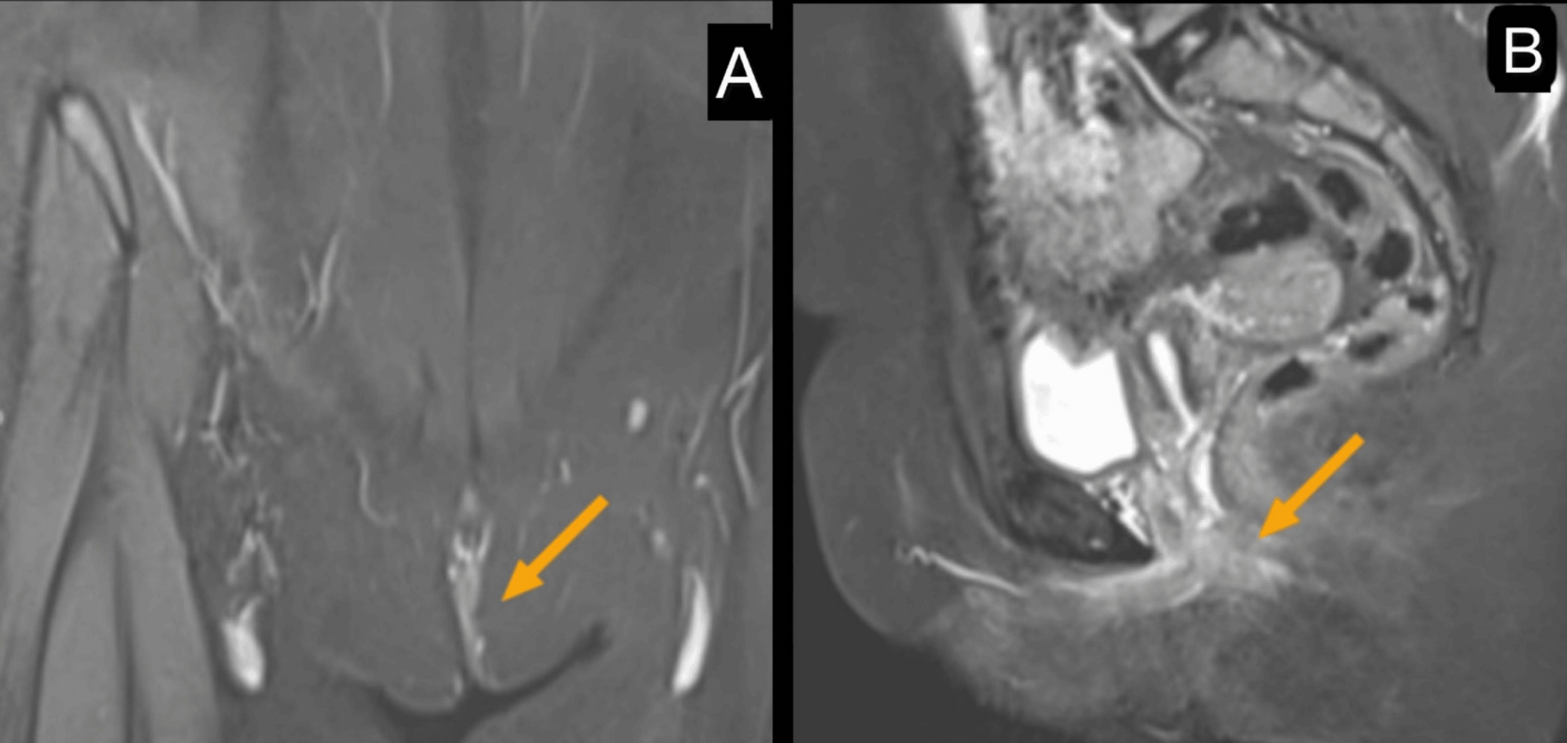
MRI fistulogram shows a curvilinear sinus tract with surrounding fibrotic changes in the subcutaneous plane of the right perineum (yellow arrow) (A) and no evidence of an abscess, mass formation, or communication with adjacent structures (yellow arrow) (B).
